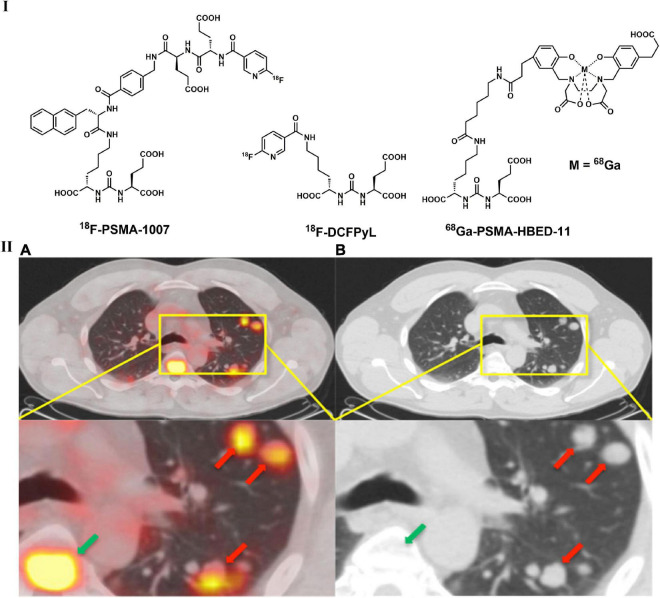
Structures of representative PSMA-targeted PET tracers (I) and PET/CT (A) and unenhanced CT (B) images of ^68^Ga-PSMA-HBED-11 in patient with with a recurrent prostate acinar adenocarcinoma and lung metastases (II).
